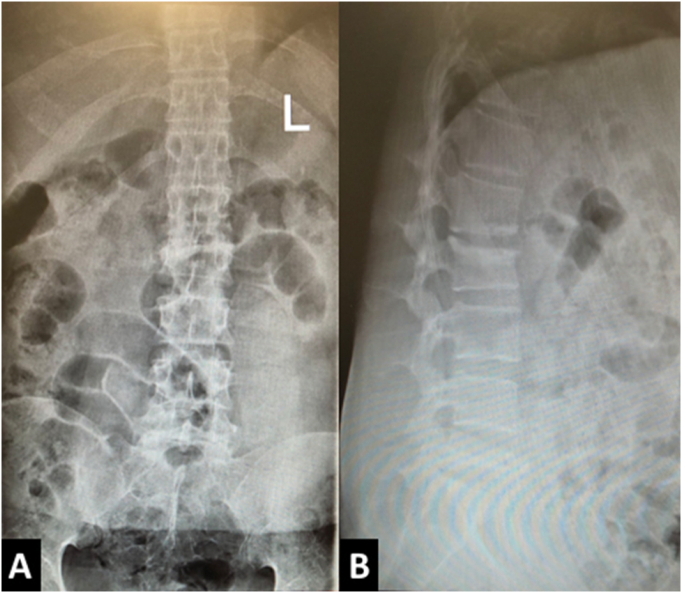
Plain anteroposterior and lateral radiographs of the lumbosacral area showing L2 compression, L5 burst fracture and intervertebral vacuum cleft sign.
